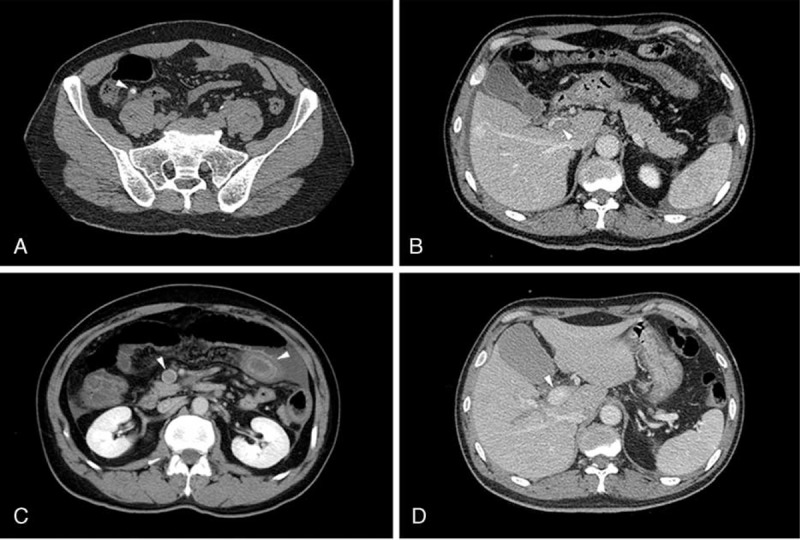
(A) An enlarged and thick-walled appendix with fecalith inside (arrows) at the level of the lower abdomen. Enhanced CT scan indicated visible thrombus with defects in the lumen of PV (B) and SMV (C), the thickened wall of small intestine indicating ischemia (C). (D) The portomesenteric veins thrombosis diminished 3 months after treatment. CT = computed tomography; PV = portal vein; SMV = superior mesenteric vein.
